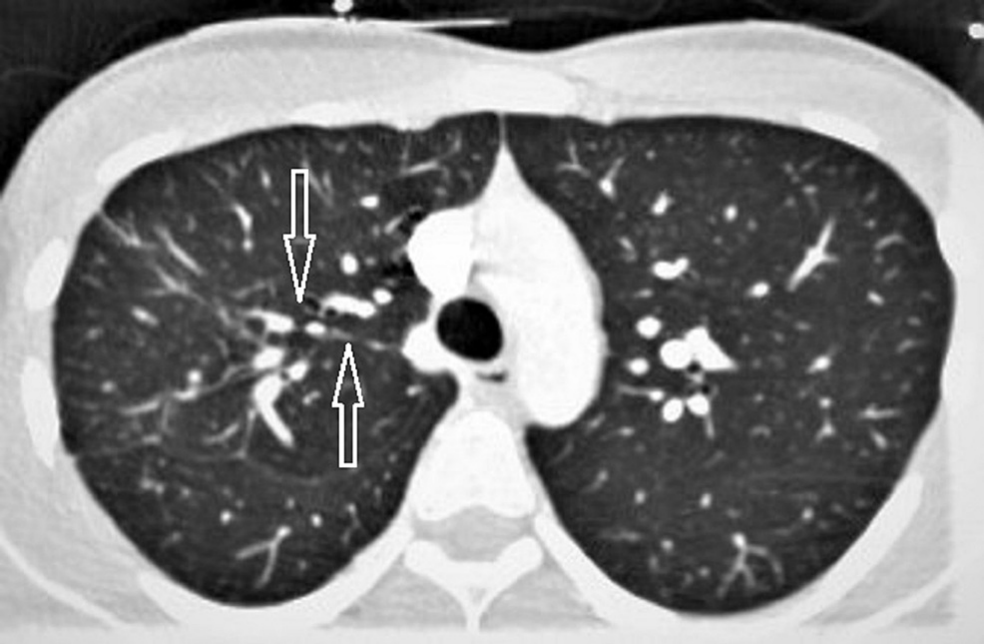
CTA showing the pulmonary emboli in the right upper lobe apical segmental and subsegmental branch of the right pulmonary artery (arrows)
